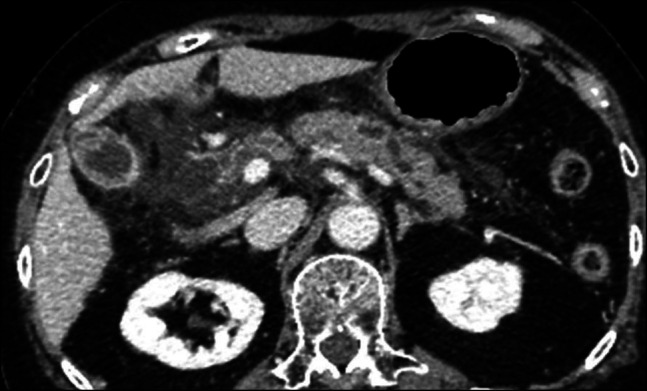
Contrast-enhanced computed tomography. Mild dilation of the distal pancreatic duct, pancreatic swelling, and increased peripancreatic fat stranding were observed and were related to the serous neoplasm.
